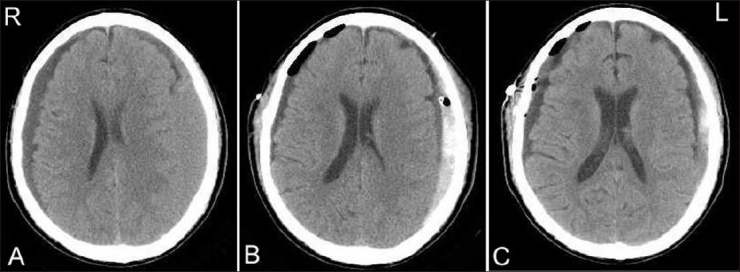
CT images of case 1. (A) CT scan on admission showing bilateral CSH. (B) CT scan 3 months after first surgery, showing recurrence of left CSH. (C) CT scan 2 months after second surgery, showing recurrence of left CSH
